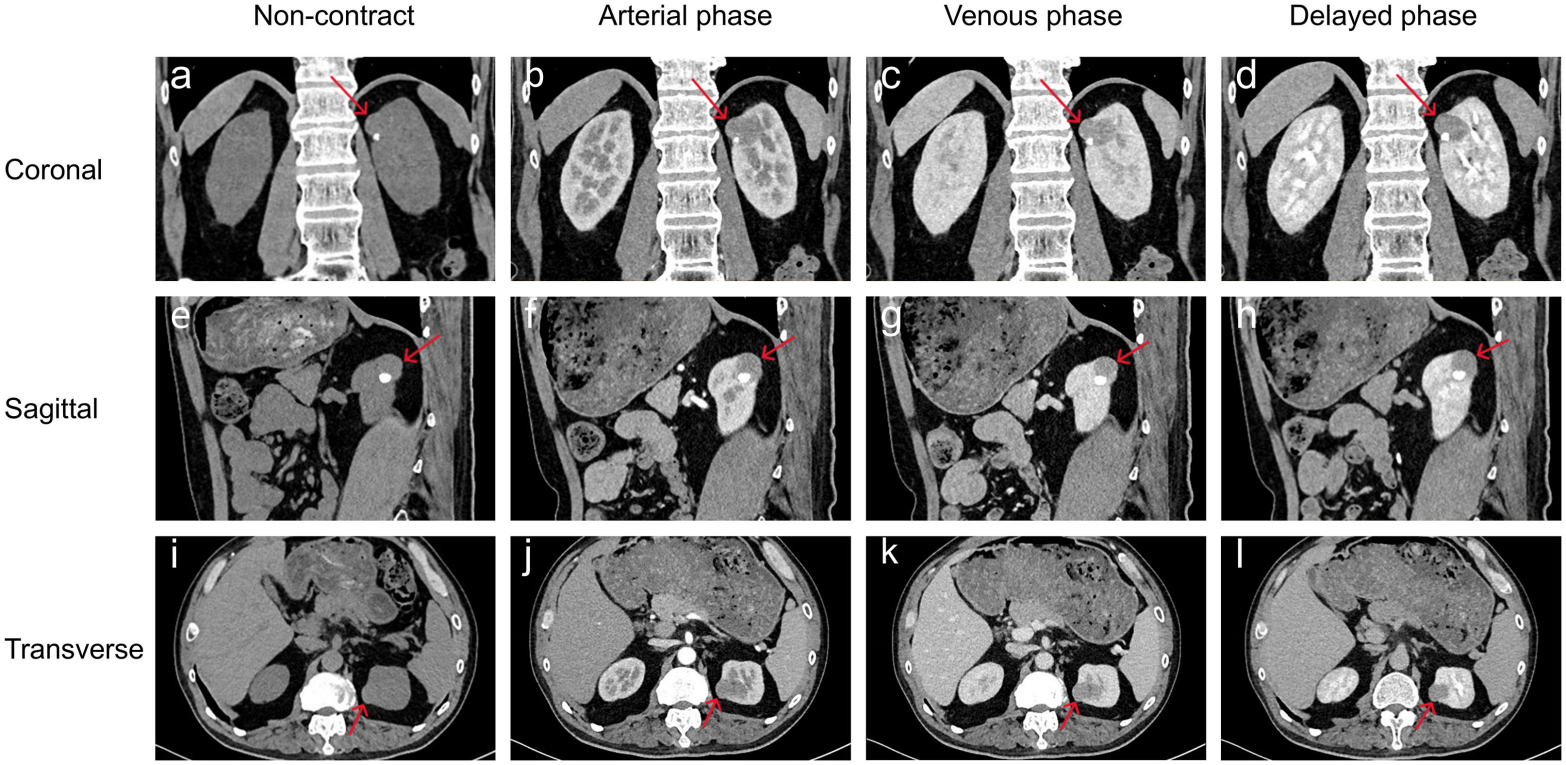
Preoperative renal CT plain scan and enhanced scan. (a-l) Multiple nodular iso-low density shadows in the left kidney, locally protruding from the outline of the kidney, and patchy calcification shadow in the left renal parenchyma. Three-phase dynamic contrast-enhanced scans revealed well-defined lesion boundaries, with mild inhomogeneous enhancement in the medial upper pole lesions of the left kidney and the rest showed no enhancement.
